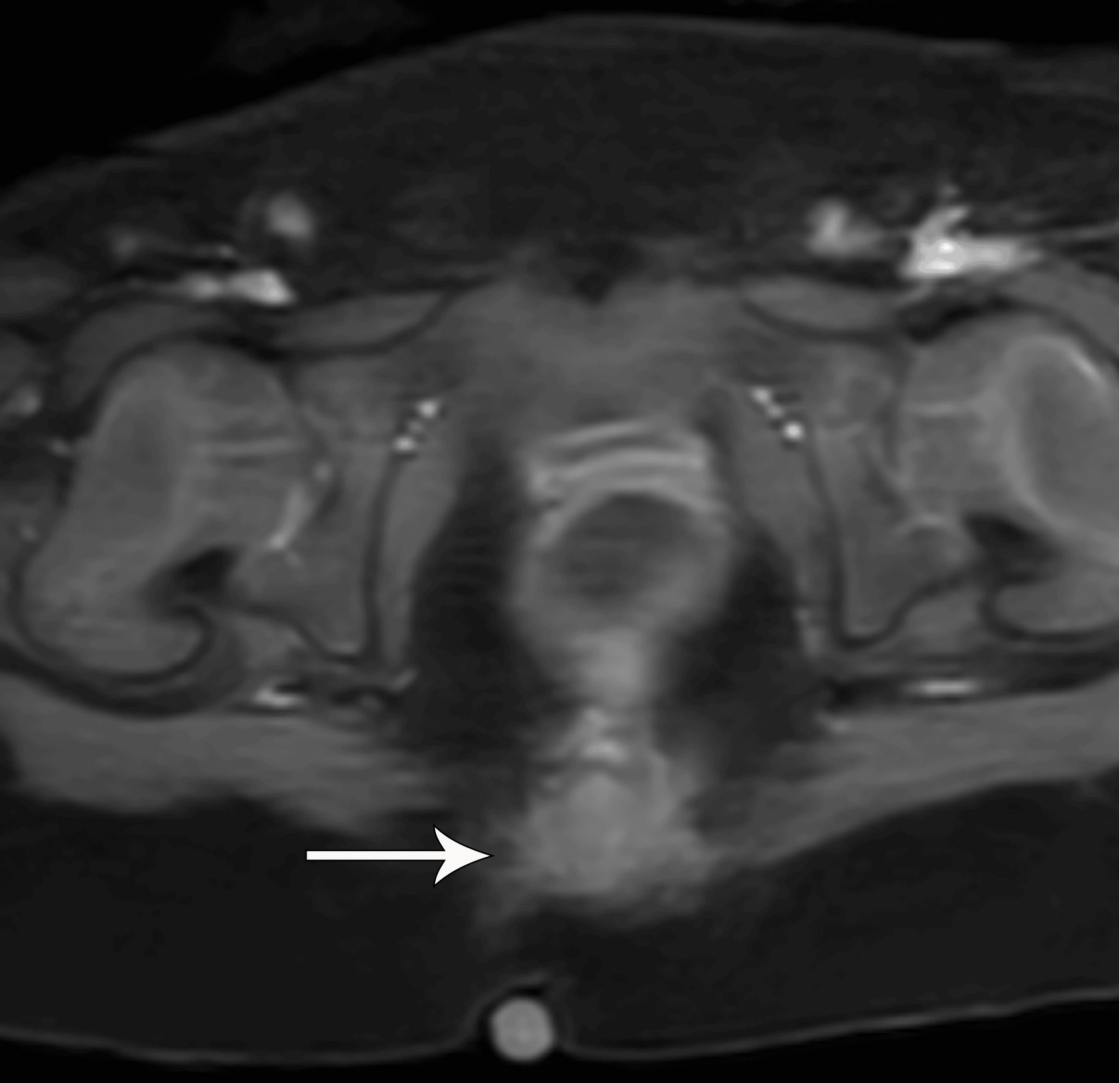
Post-contrast T1-weighted fat-saturated axial MRI image showing homogenous enhancement (white arrow)
